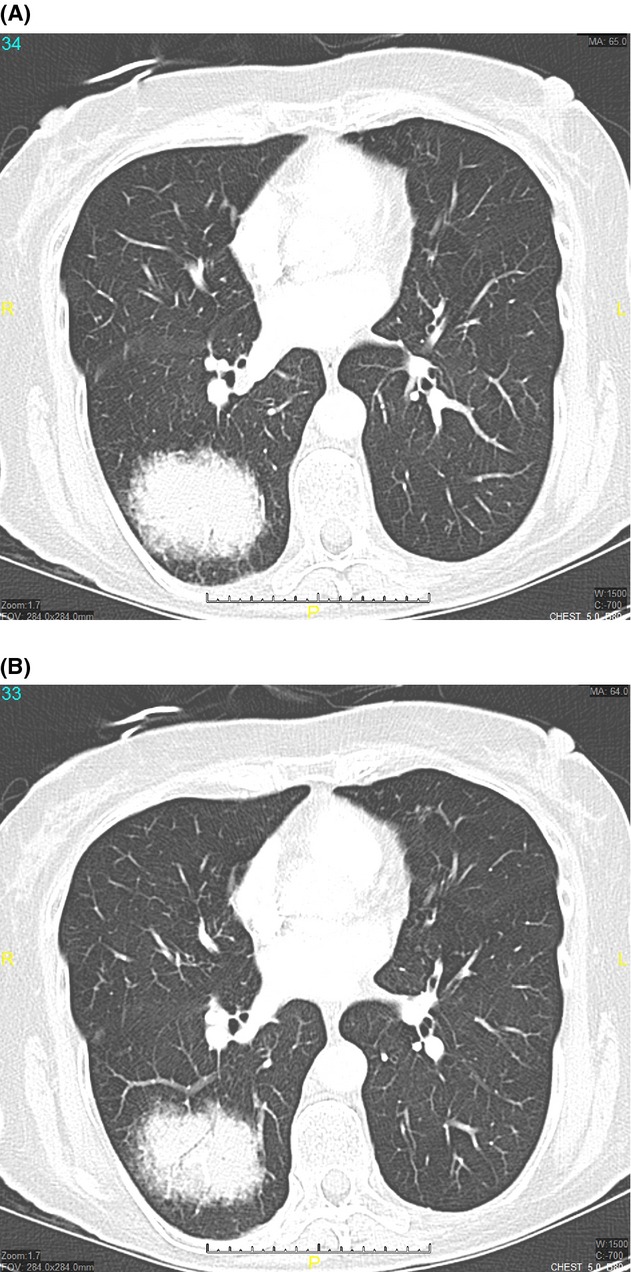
(A and B) Computed tomography (CT) scan cross sections showing round consolidation with air bronchogram.
